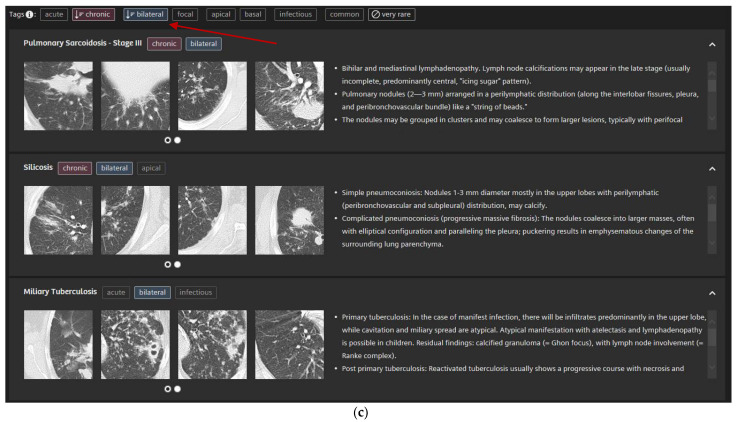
5 mm transversal lung window CT of a 51-year-old female patient with pulmonary sarcoidosis and corresponding CBIR user interface: Participants were shown the corresponding 5 mm transversal lung window CTs for each case. They then drew a ROI into the area with their supposed dominant pattern (a). Readers were then presented the CBIR results (b) and could select high-level semantic features (e.g., chronic, bilateral) to filter retrieval results (c).
